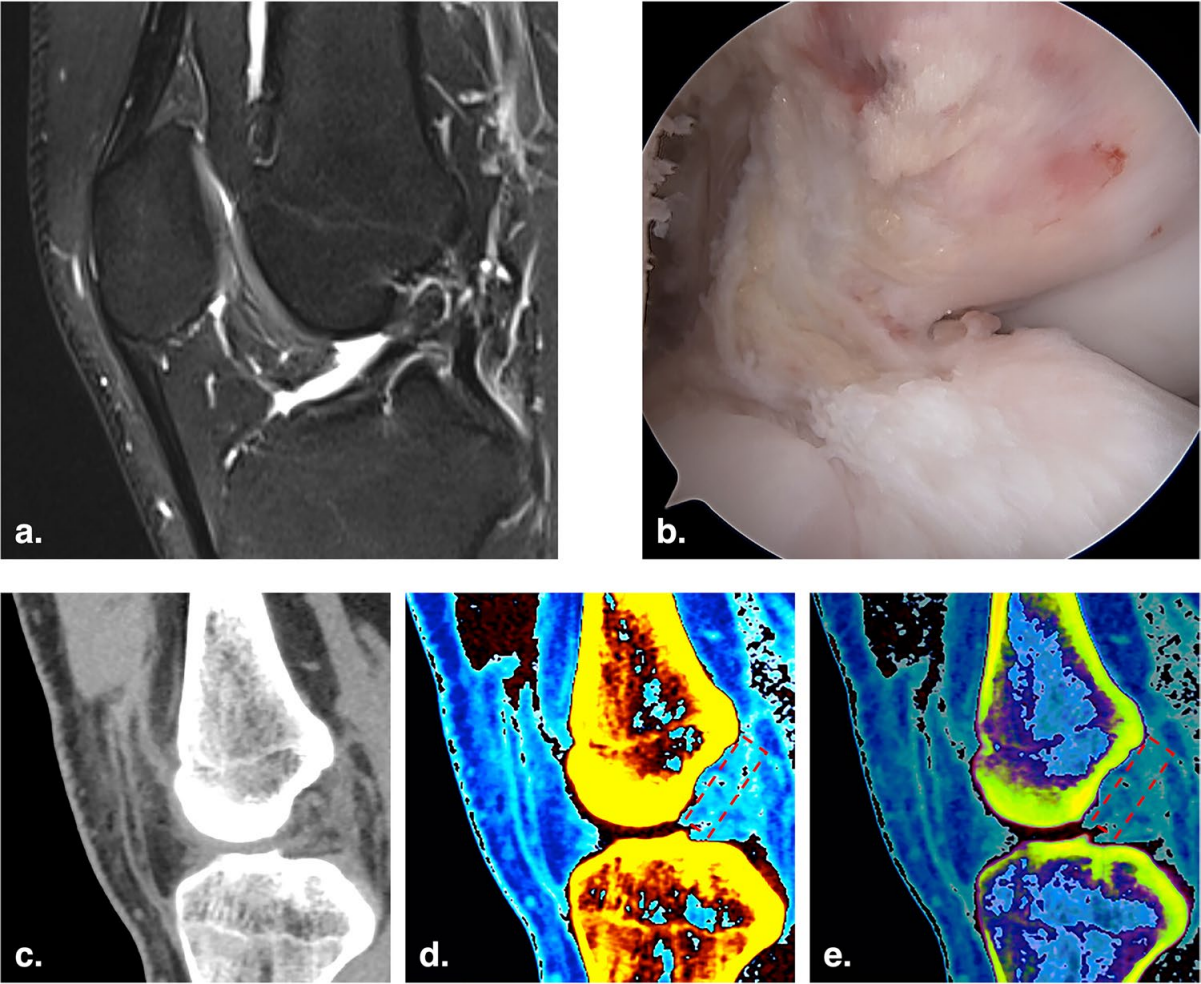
Images of a 22-year-old man with right ACL rupture. a Sagittal T2-weighted fat-saturated MRI image of the right knee. b Arthroscopic image of the torn right ACL. c Monochromatic DECT image with normal mode (mixed keV) on the oblique sagittal plane. d and e Color-coded DECT images with mono + mode (80 keV) and Rho/Z mode (mixed keV) on the oblique sagittal plane, respectively. The torn ACL was not colored in the anatomic position (red dotted rectangle)
